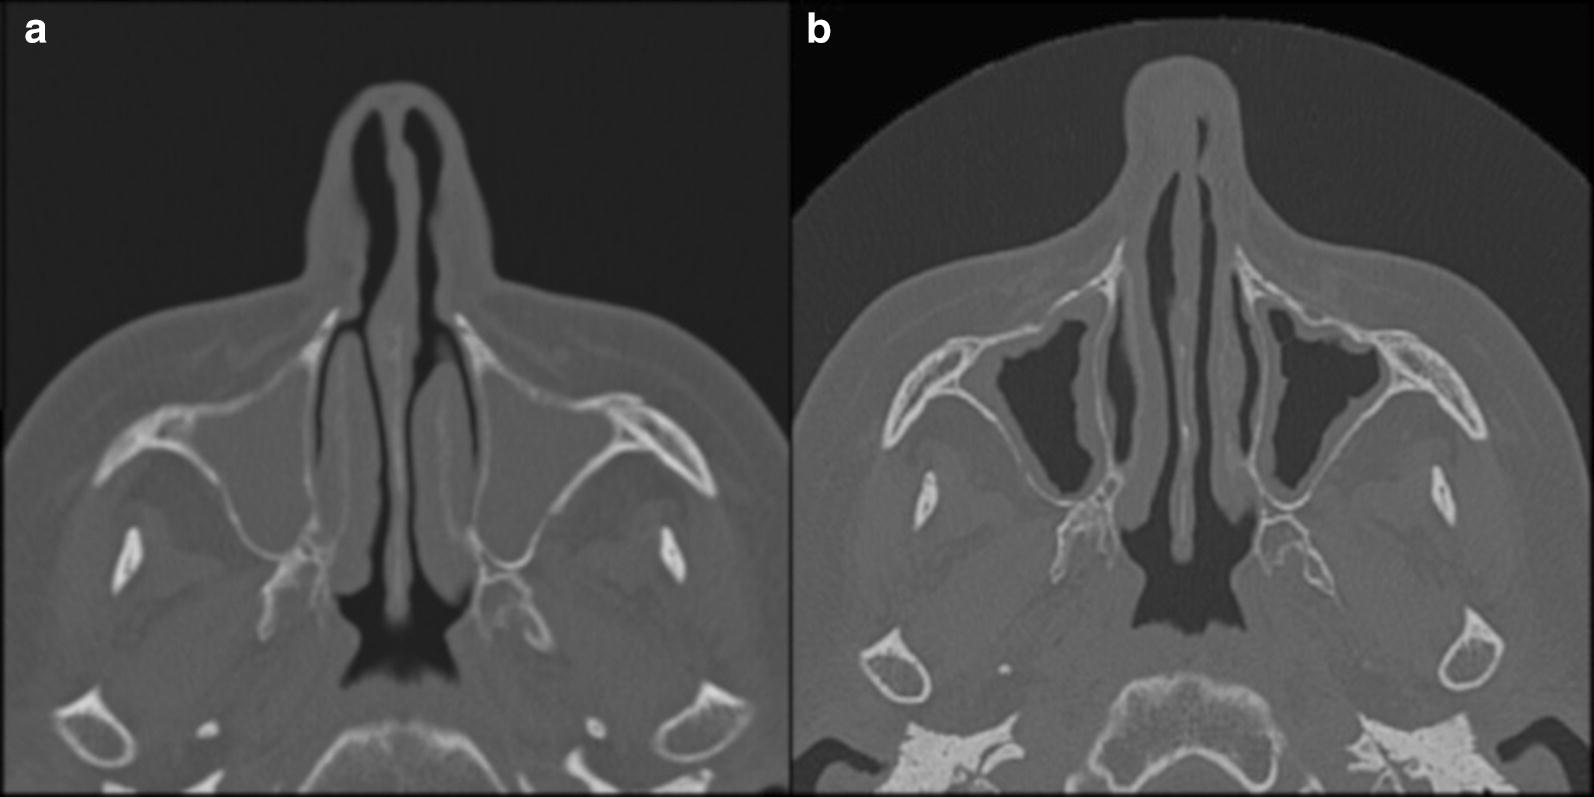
Axial CT comparison before and after therapy: paranasal sinuses CT in axial projection at t0: bilateral engagement of maxillary sinuses (a), and at T1 CT: clear improvement in the maxillary sinuses which show only slight mucosal hypertrophy (b)
